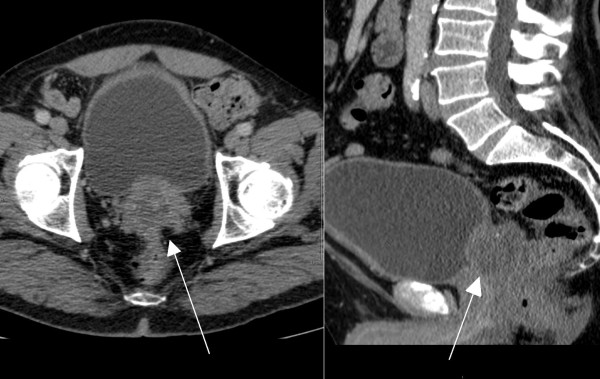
Computed tomography (CT) images demonstrating the prostate with metastatic invasion.
